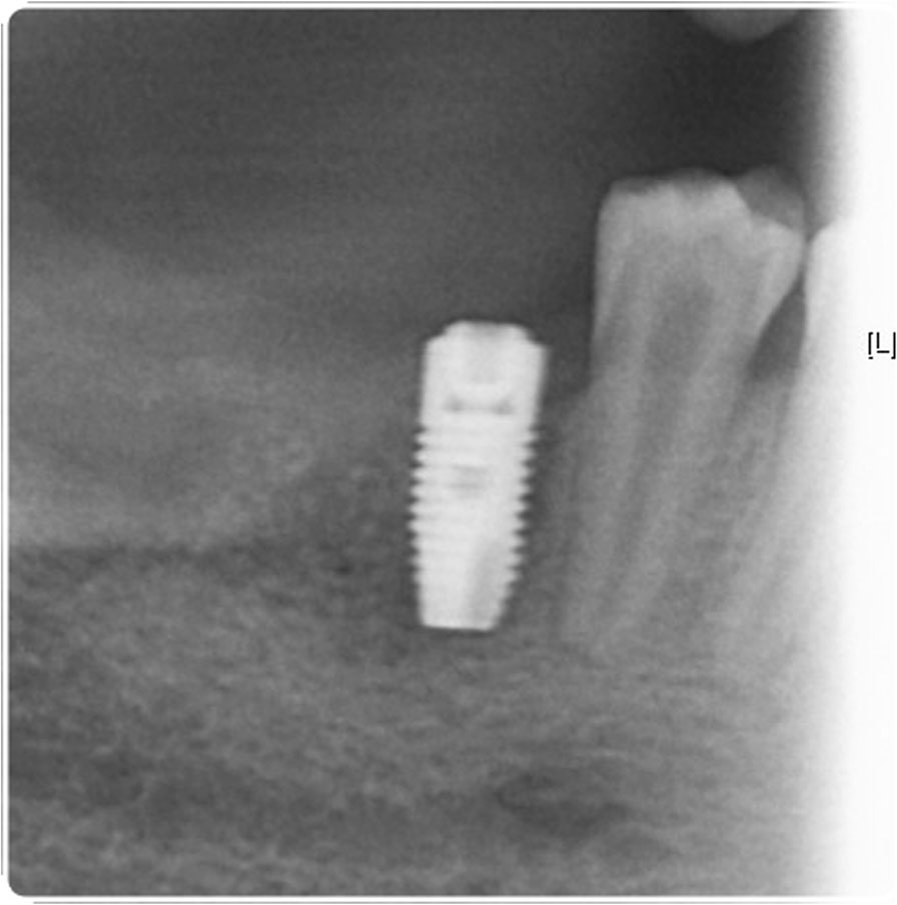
Most recent fixture radiograph. Radiograph of fixture in 46 position
